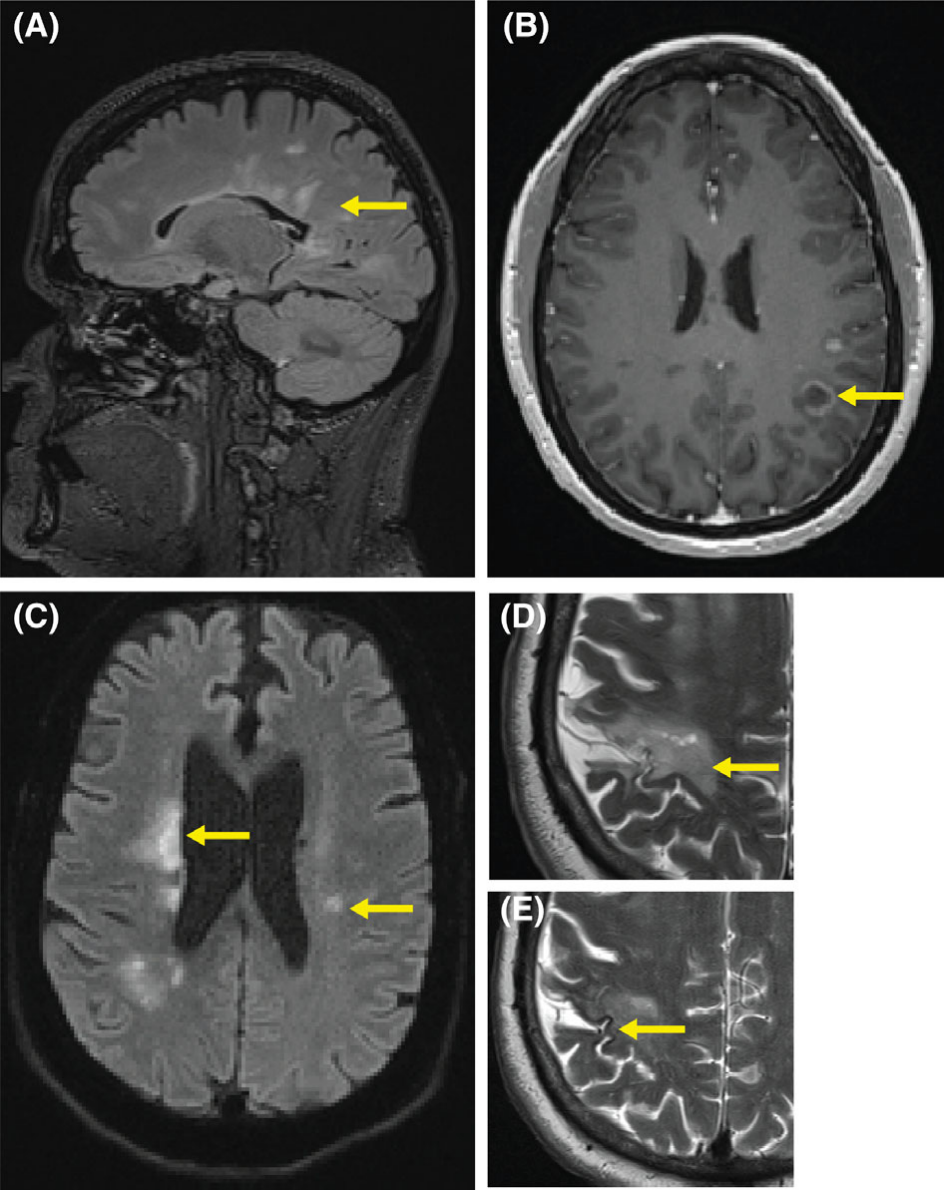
MRI features of non‐MOGAD diagnoses. (A) Perpendicular, periventricular T2 FLAIR hyperintensity (Dawson's Fingers, arrow) pathognomonic for multiple sclerosis. (B) T1 postgadolinium enhancement in an incomplete ring pattern (arrow) in the left parietal lobe consistent with demyelination in MS. (C) Bilateral periventricular and right cortical parietal lesions demonstrating diffusion restriction of varying intensity suggests recent established infarctions of varying acuity. (D and E) 3 months after (C) demonstrates right parietal lobe cortical and subcortical T2 hyperintensity with adjacent gyriform hemosiderin deposition consistent with interval established infarct and adjacent blood product deposition, in combination, suggestive of vasculitis.
